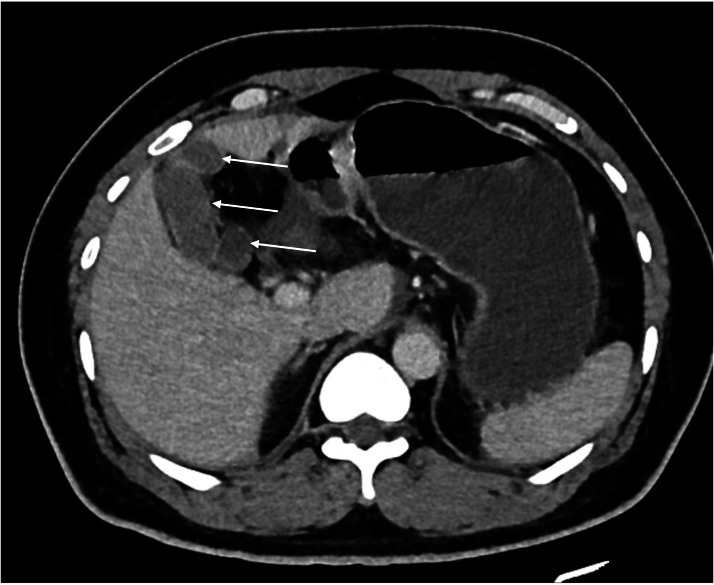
Axial portal venous phase abdomeninal CT of the same patient at the level of T11 taken 10 months prior from the MRCP shows 3 distinct lobes of the gallbladder (arrows) draining to the single cystic duct through the medial lobe.
